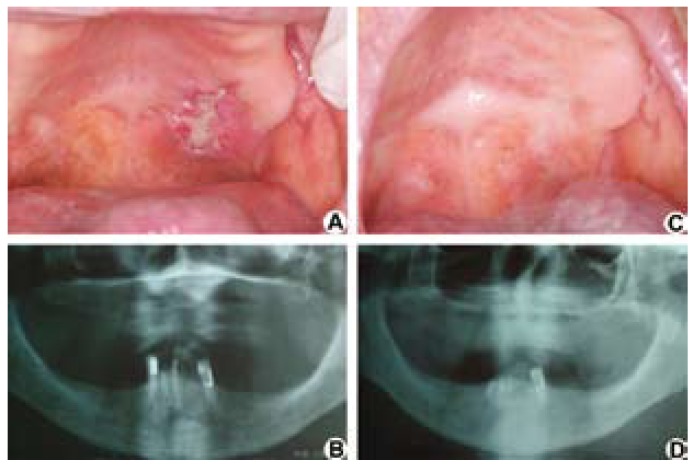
A) Initial clinical image. An ulcer of raised and irregular edges could be observed on the border of the hard and soft palates. B) Initial panoramic radiographshowed no bone changes. C) Clinical image and D) panoramic radiograph after thirty-one months of diagnosis and treatment, in which the lesion’s total clinical resolution could be observed.
